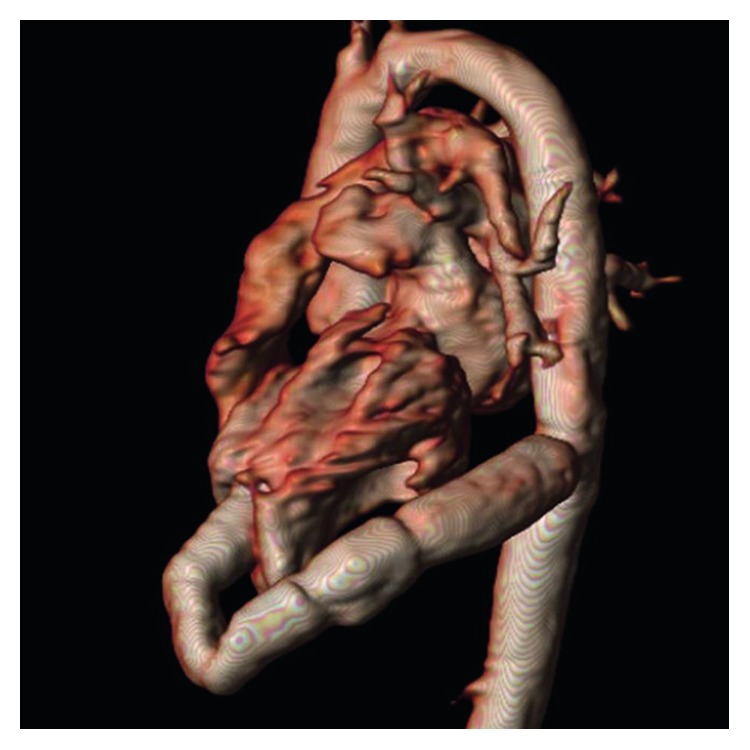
MRI shows a conduit from the apex of the left ventricle to the descending aorta.
